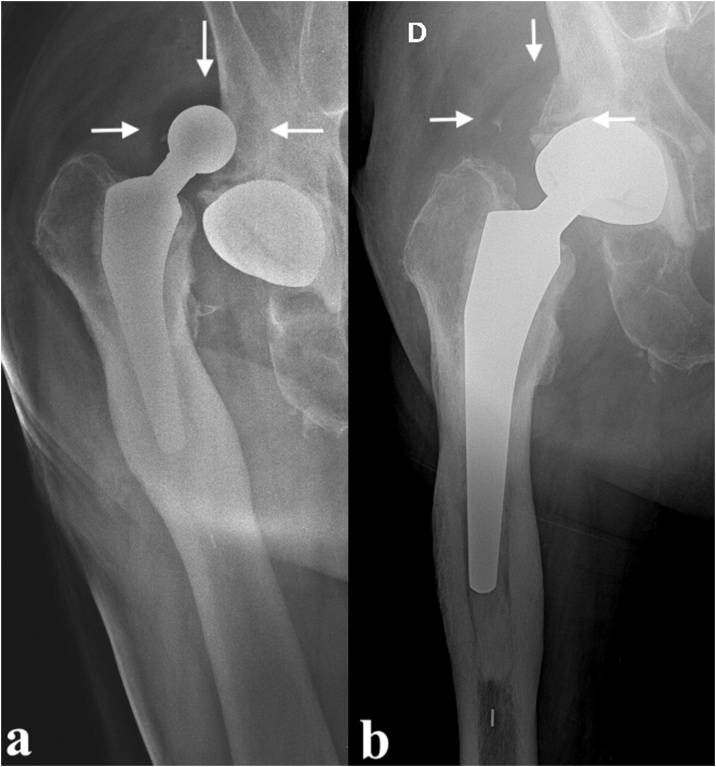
X-rays takenbefore (a) and after (b) closed reduction (the arrows point to the “bubble sign”).
